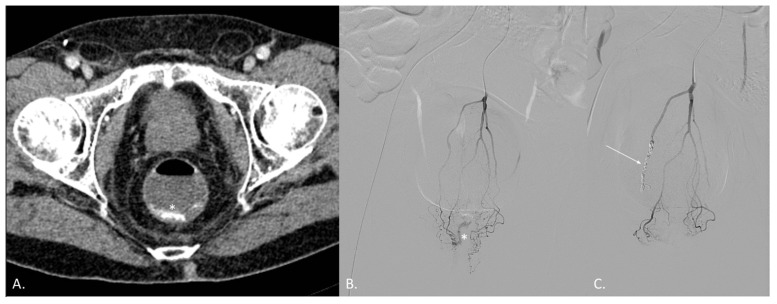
(A–C) CT angiography (CTA) and digital subtraction angiography (DSA) of a 68-year-old male patient known for radiation-induced proctitis after prostate cancer treatment. (A) CTA demonstrates active endoluminal bleeding (*) from the superior hemorrhoidal artery (SHA). (B) DSA obtained with catheterization of the inferior mesenteric artery (IMA) confirms CTA findings. (C) DSA obtained with catheterization of the IMA after coil embolization of the SHA (arrow). No active bleeding evidence.
